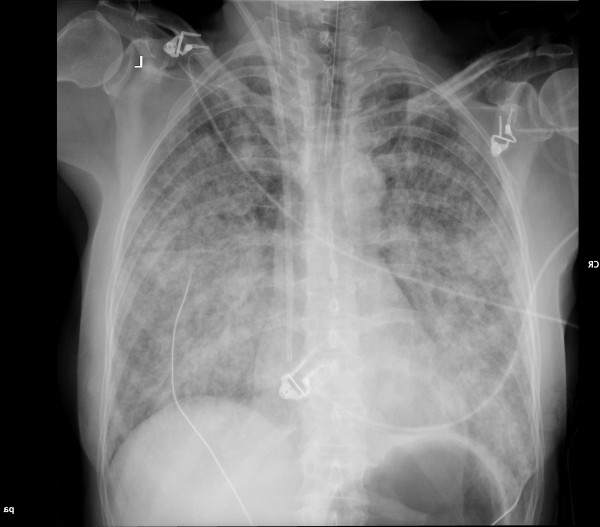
X-ray of the chest of the patient in the presented case showing bilaterally pulmonary oedema.
